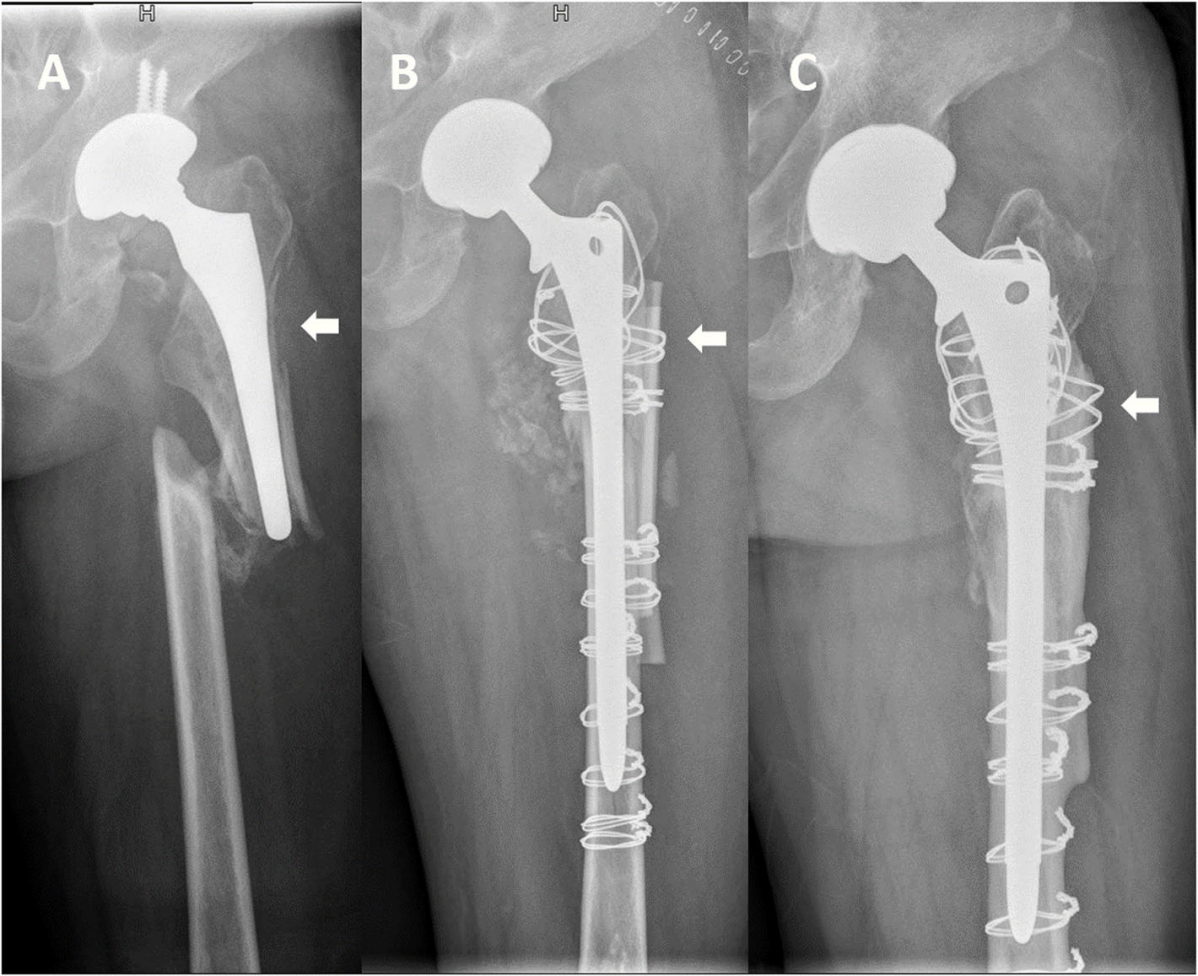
a Preoperative radiographs of a Vancouver-type B3 periprosthetic femoral fracture. b Radiograph at postoperative day 1. Periprosthetic femoral fracture was treated with extensively porous-coated stem and cortical strut allografts. c At 10-year follow-up, no subsidence and radiolucent lines was identified and the stem was considered to be bone ingrowth stable. Bone restoration of bone defect area (white arrow) was observed. Cortical strut allografts incorporated to the host bone successfully and the resorption of cortical strut was assessed as mild
